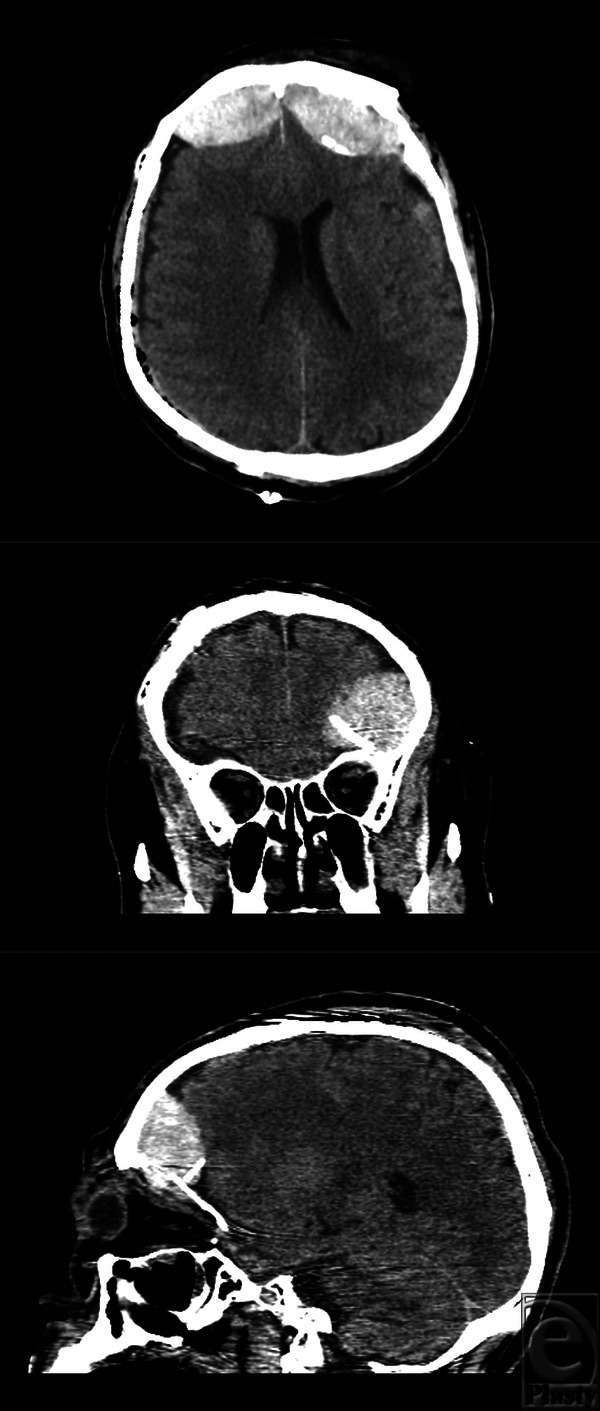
Brain computed tomography after the first operation at the local hospital. Upper: axial view. Middle: coronal view. Lower: sagittal view. Epidural hematomas are seen on both anterior frontal convexities. A displaced orbital roof fracture segment is seen.
